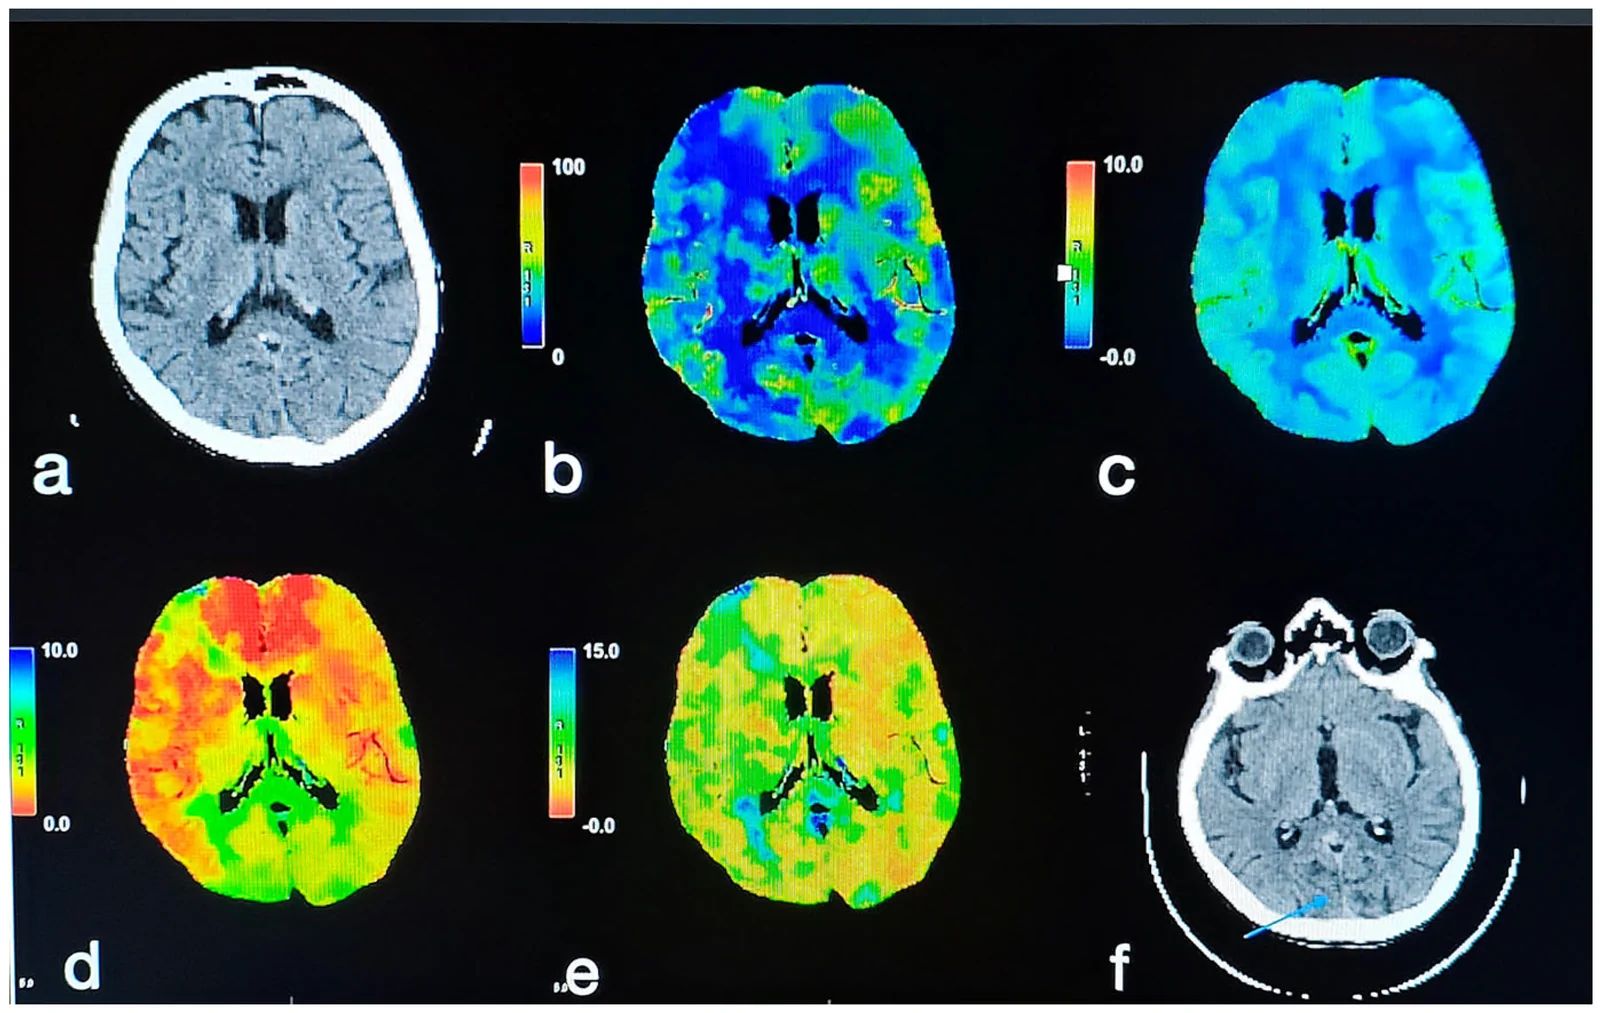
An 83-year-old female patient from Hospital “Saint Sava”, with dizziness and right-sided weakness. NCCT without pathological changes, ASPECT 10 (a). CTP representation showing CBF (b), CBV (c), MTT (d) and Tmax (e) without perfusion deficit. Follow-up NCCT after 24 h shows an acute ischemic lesion on the left occipital lobe (blue arrow) (f).
